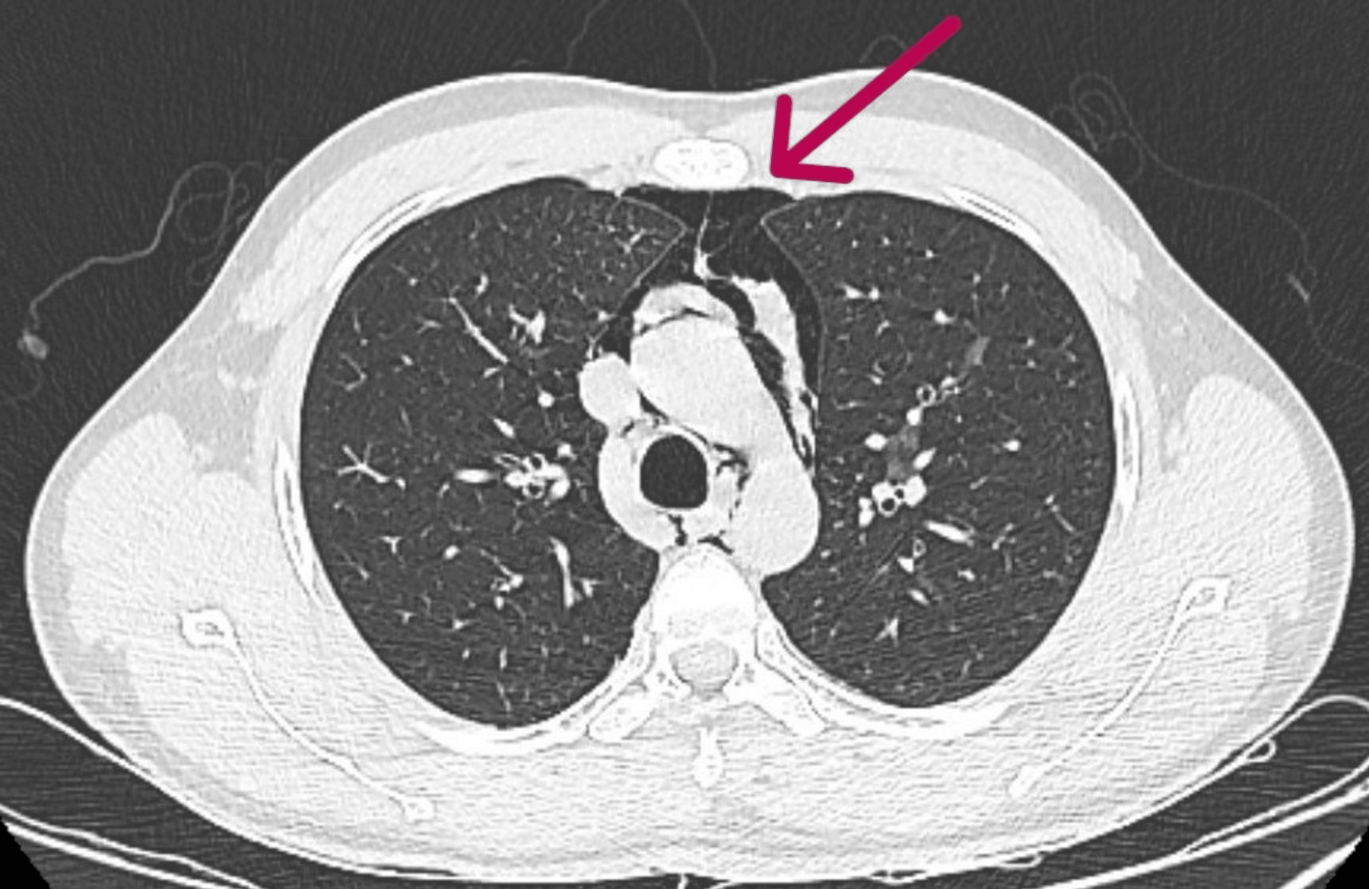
Chest CT showing air pockets around the great vessels and in the pericardium (taken July 24, 2022).
